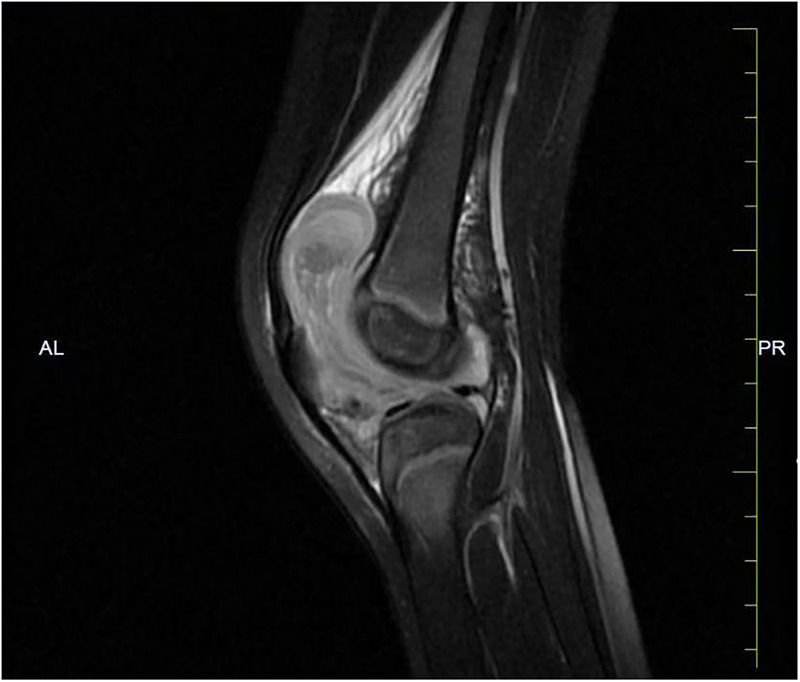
MRI of the left knee showing suprapatellar and joint cavity effusion with synovial hypertrophy and associated soft tissue swelling on T2-FSE imaging.
